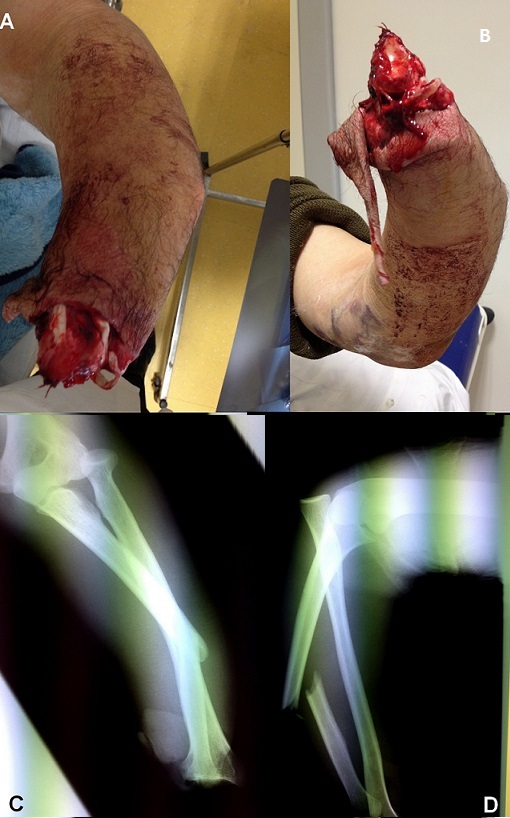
(A and B) clinical features in patient at admission showing wrist disarticulation with a deformition of the elbow and forearm; (C and D) radiography objectified a Monteggia fracture-dislocation with a radiocarpal amputation of wrist-joint
